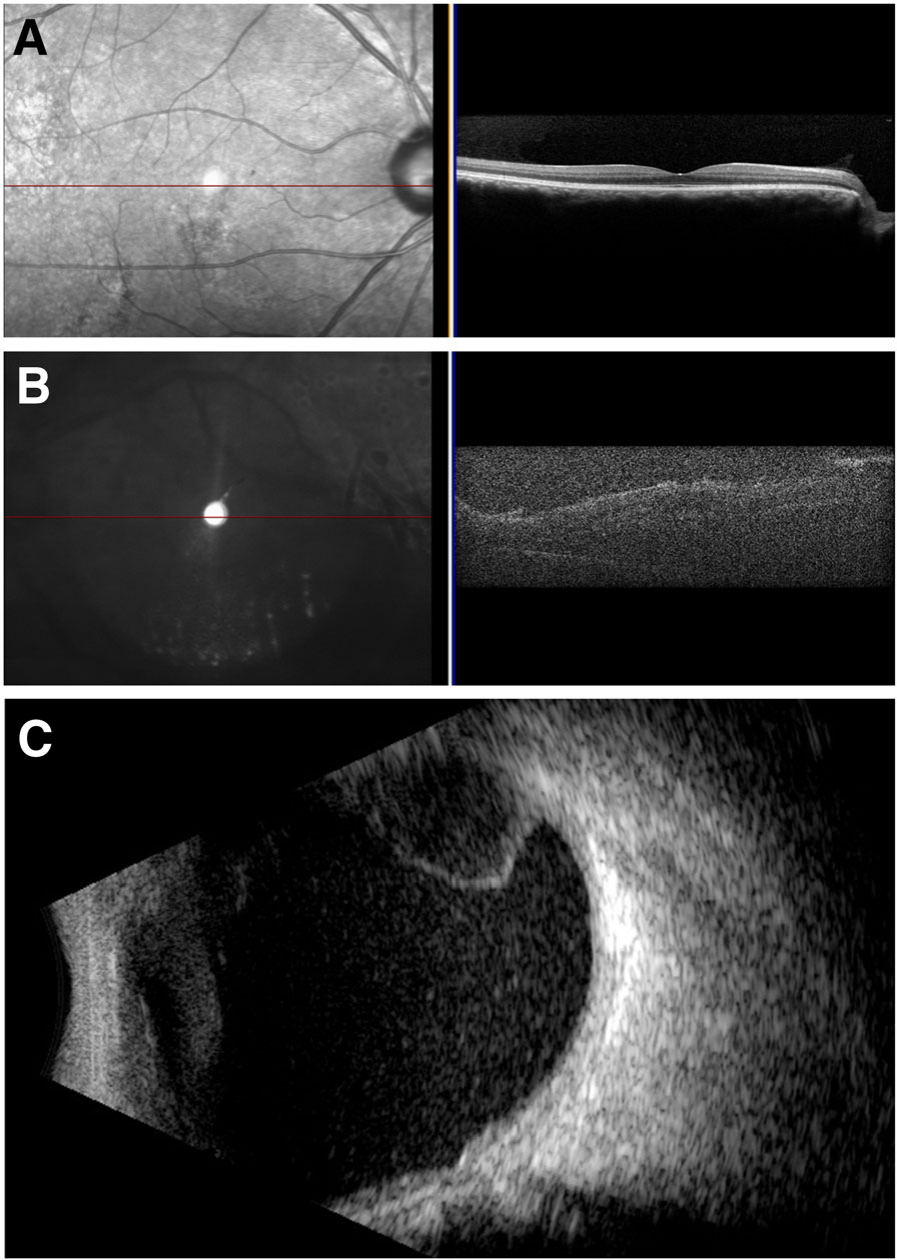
Optical coherence tomography and B-scan ultrasound of both eyes. Optical coherence tomography (OCT) of the right eye was normal (a). In the left eye, OCT demonstrated subretinal fluid and a limited quality scan due to vitreous haze (b). B-scan ultrasound noted a serous retinal detachment OS without any retinal breaks (c)
